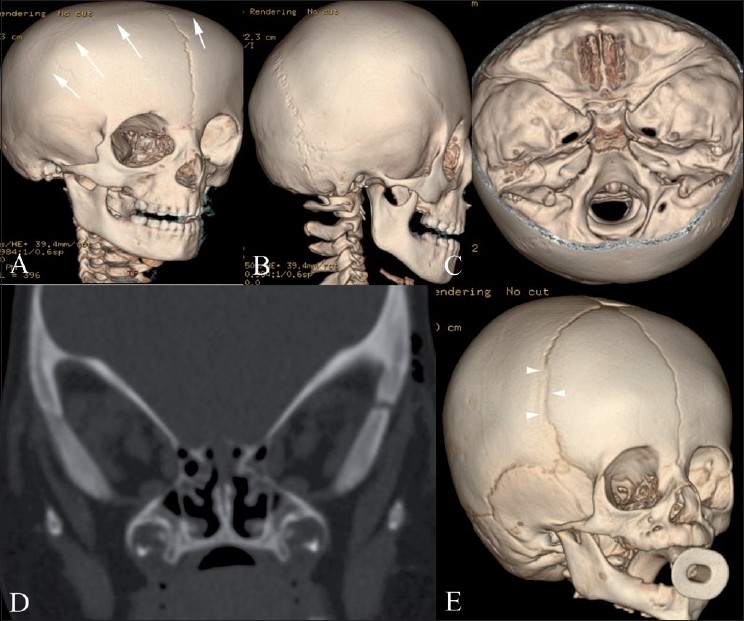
Bilateral (A-D) and unilateral partial (E) coronal synostosis 3DCT volume rendered images (A-C, E) and coronal CT scan (D). There is complete fusion of the coronal suture (white arrows) with a prominent frontal bone and flattened occiput. Coronal reconstruction (D) demonstrates prominent bilateral elliptical orbits, known as the “harlequin eye” deformity. Note the early partial fusion of the right coronal suture (arrowheads in E)
